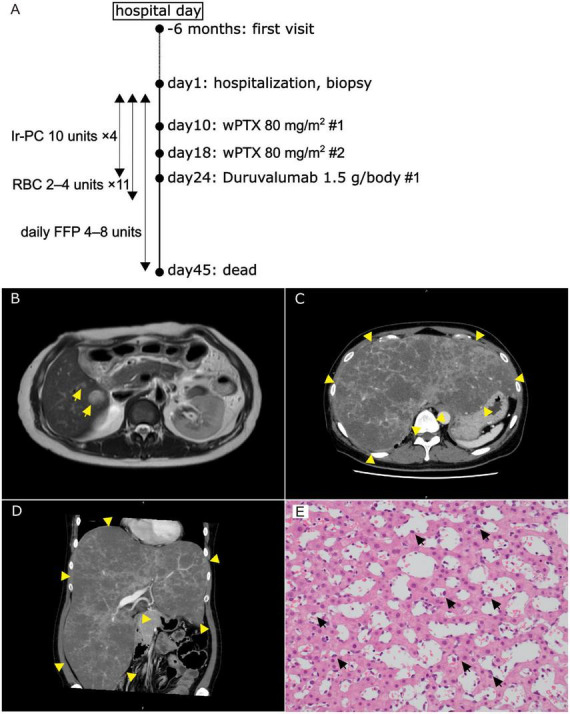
Radiology imaging and biopsy findings of the lesion. (A) Timeline of the clinical course of the patient, including percutaneous liver biopsy and treatments. (B) An initial abdominal MRI reveals multiple T2-hyperintense lesions. This slice shows two lesions (arrows). (C,D) Arterial-phase contrast-enhanced computed tomography (CT) performed 6 months after the initial MRI shows multiple low-density areas (32 HU) of varying sizes diffusely involving the entire liver in the axial [(C); maximum liver diameter, 272 mm] and coronal[(D); maximum liver diameter, 347 mm] planes (arrowheads). (E) Biopsy findings show dilated sinusoids lined by enlarged endothelial cells with minimal atypia (arrows). wPTX, weekly paclitaxel; Ir-PC, irradiated platelet concentrate; RBC, red blood cell; FFP, fresh frozen plasma.
